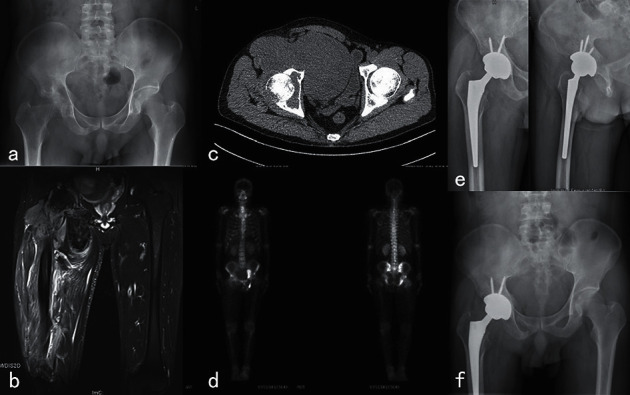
40-year-old male, giant cell tumor of the tendon sheath in sections I and II of right hemipelvis. First-stage surgery of most of tumor resection and exploration of iliac vessels and the sciatic nerve. Second-stage surgery of resection of residual tumor, total hip arthroplasty, autologous bone graft, and allograft acetabular reconstruction. (a) Preoperative X-ray, (b) preoperative CT scan, (c) preoperative MRI, (d) preoperative bone scan, (e) X-ray at 6 months after an operation on anteroposterior and lateral position, and (f) X-ray at 12 months after the operation.
